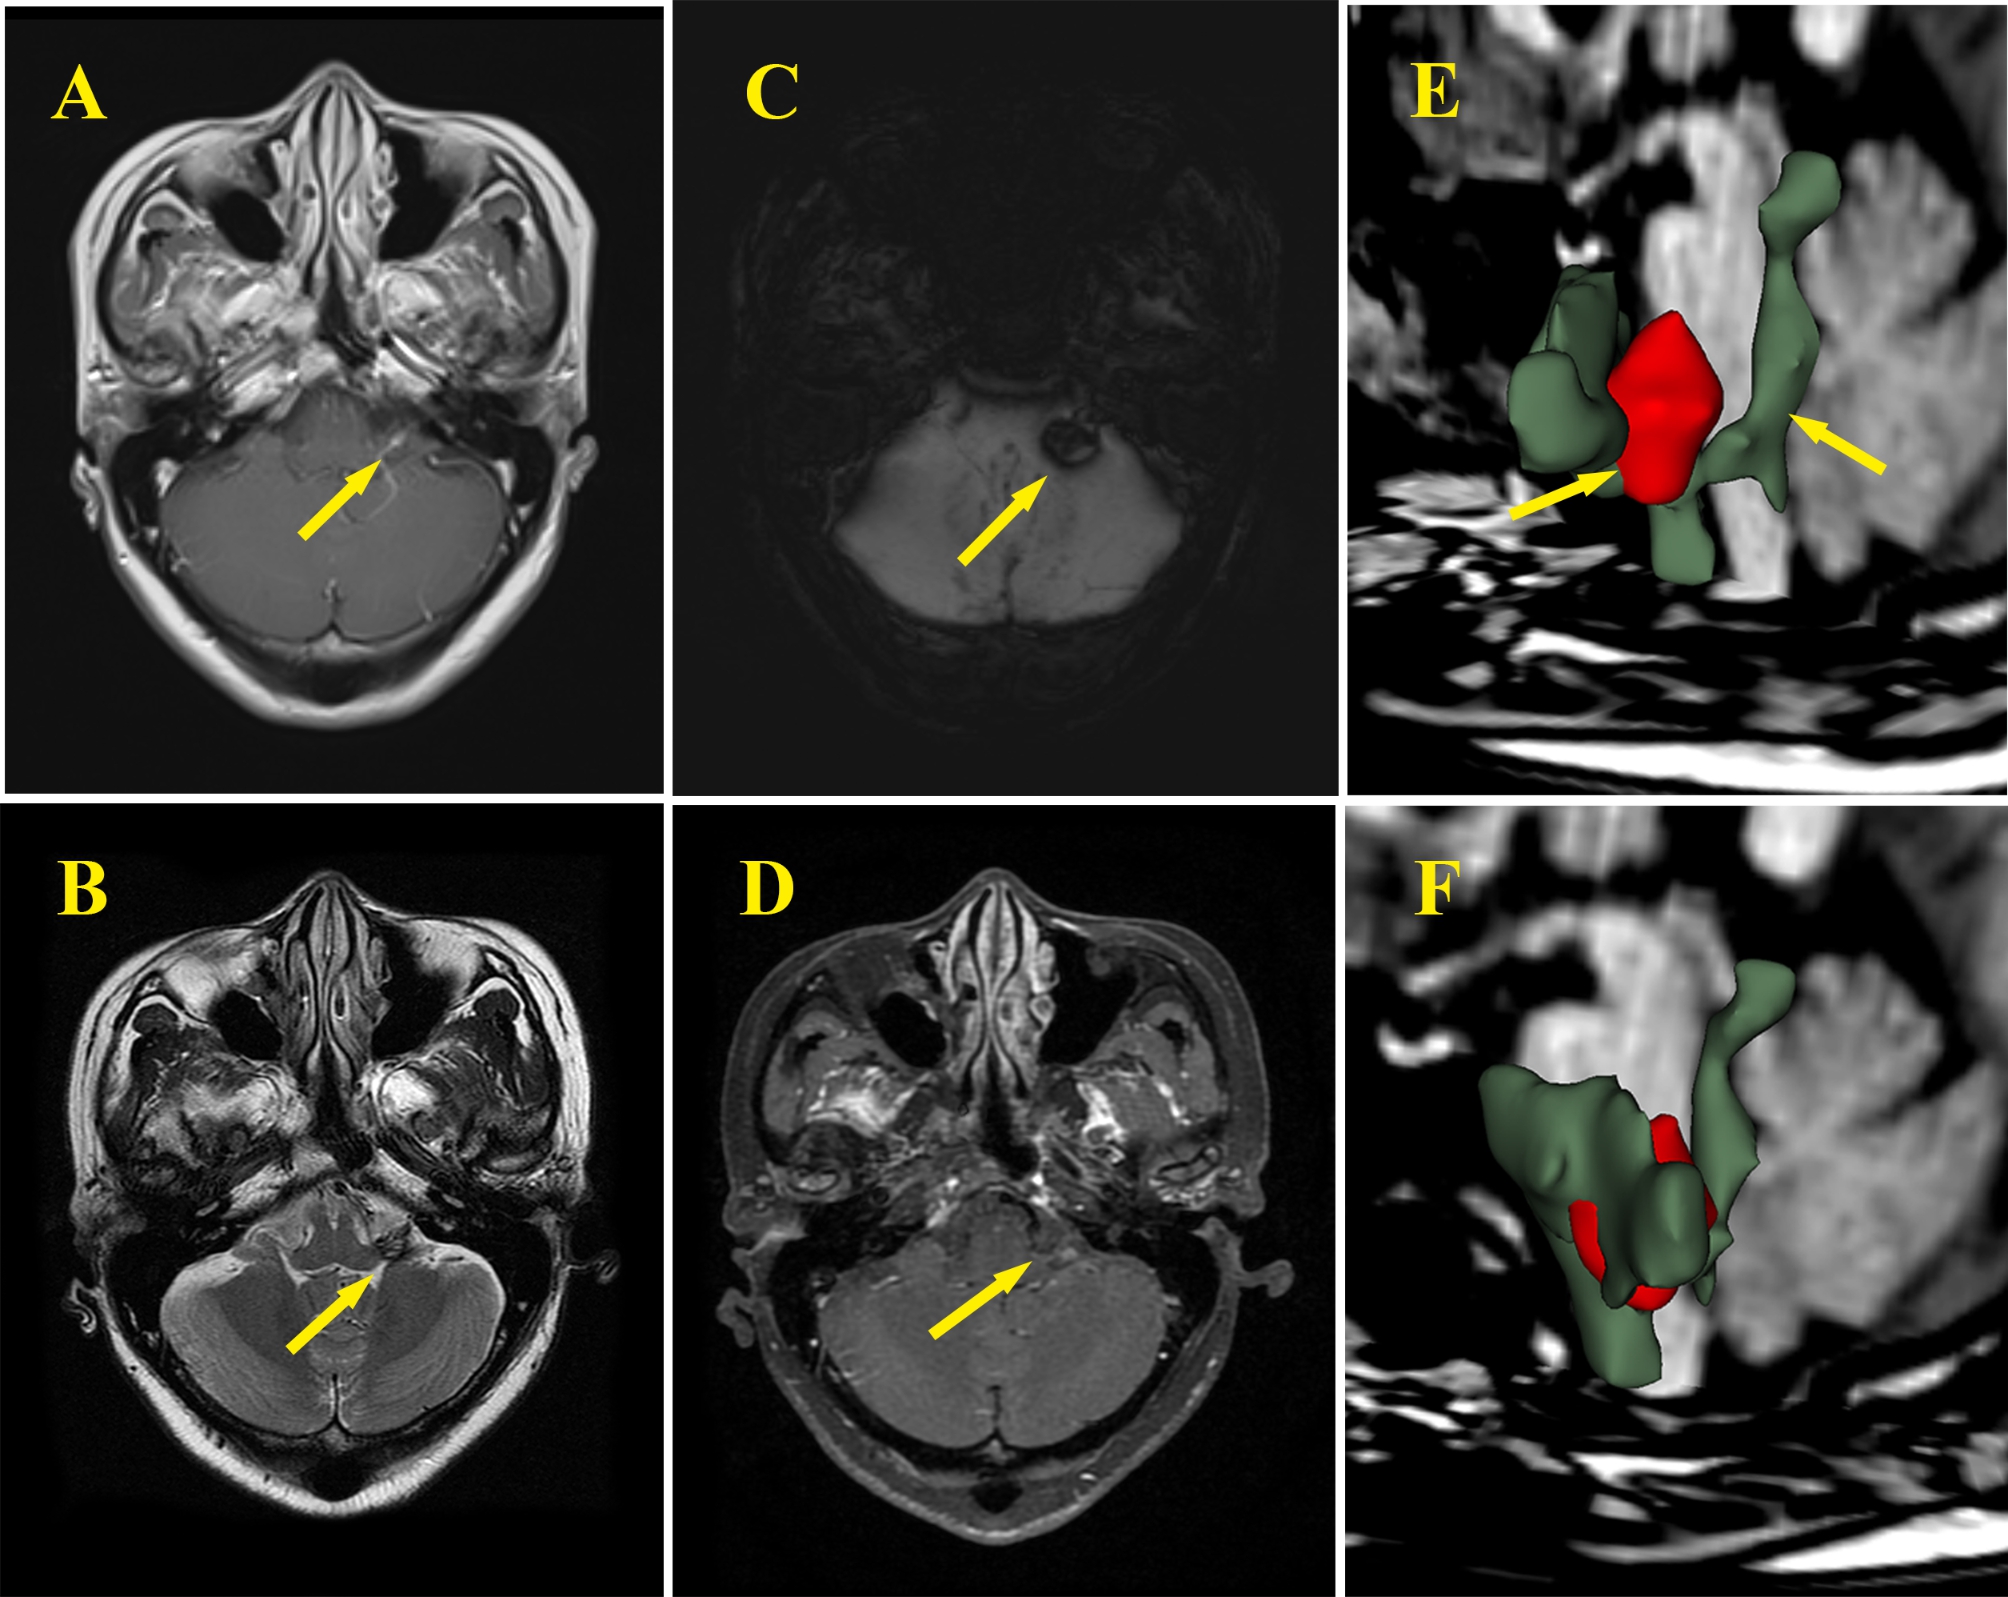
The MR image showed the lesion of the cisternal segment of the root of the left auditory nerve (A, B, C, D). The reconstruction using 3D-slicer software shows the anatomical relationship between the lesion (red) and the cisterna (green) (E, F)
